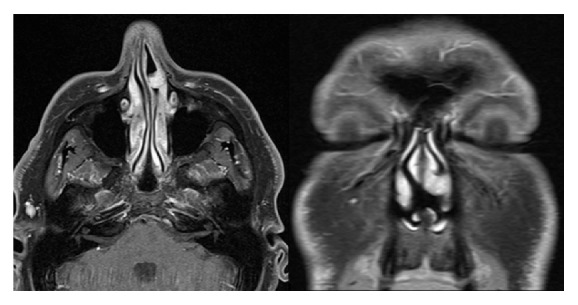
Contrast enhanced MRI, T1-weighted showing a single nodular lesion emanating from the lateral nasal wall and extending to the anterior face of the inferior nasal turbinate on the level of the piriform aperture without any sign of infiltrative growth.
